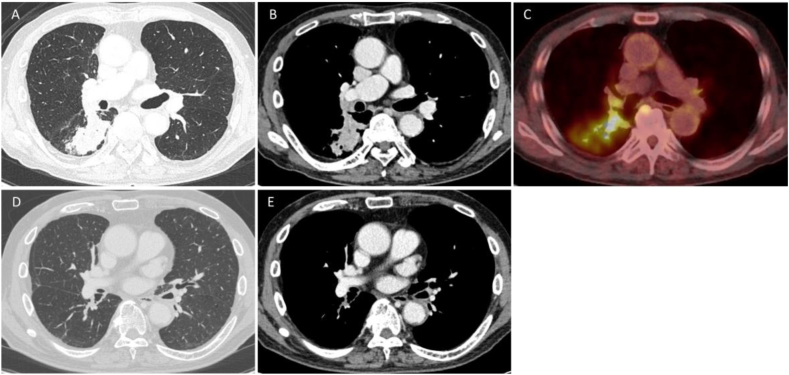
A and B: Chest computed tomography (CT) before neoadjuvant chemo-immunotherapy showing a 50-mm solid tumor in the right S6, a swollen #11s lymph node, and obstructive pneumonia peripheral to the tumor. C: Positron emission tomography (PET) before neoadjuvant chemo-immunotherapy. D and E: Chest CT after neoadjuvant chemo-immunotherapy showing near-complete disappearance of the tumor.
